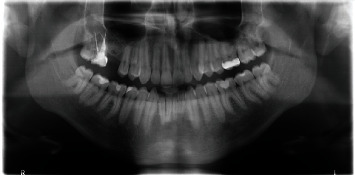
Panoramic radiograph taken immediately after sinus grafting.
